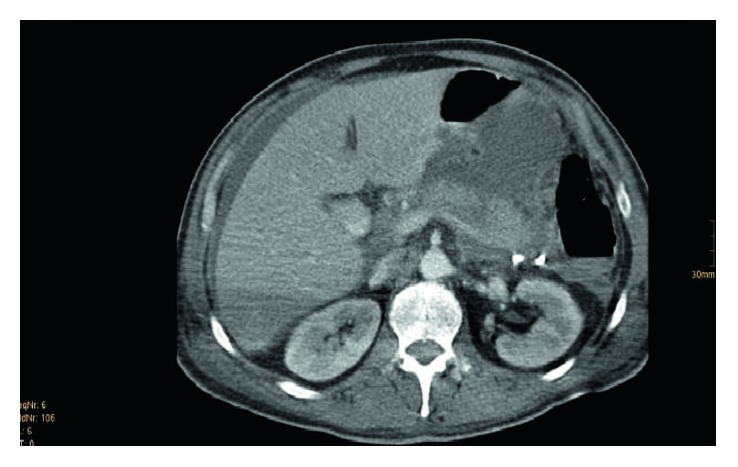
(Patient 2, scan 1) CT scan 15 days after trauma and admission after hospital referral. CT scan after the introduction of drainage from the left lateral to the retroperitoneal. The pancreas appears to be altered beside the corpus and the tail. In particular, hypointense formation in the pancreatic body is shown in the ventral part of the pancreas due to the injury. It connects with the remaining drainage. Free fluid is shown in the perihepatic and perisplenic areas.
